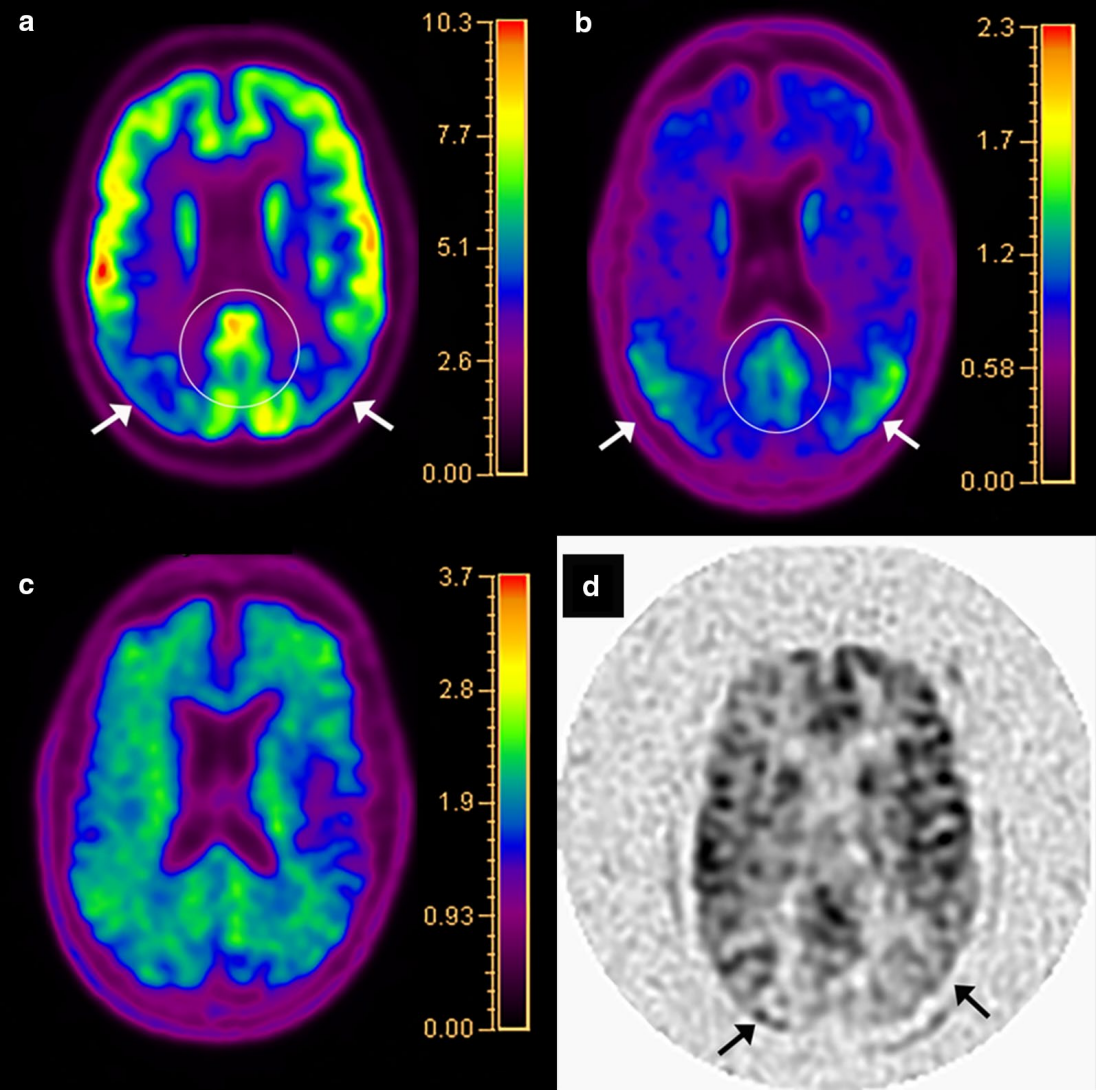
A 73-year-old man with AD, Mini Mental State Examination (MMSE) 23/30. Fluorodeoxyglucose PET/CT (a) demonstrates hypometabolism involving the bilateral parietal lobes (arrows) and precuneus and PCC (oval). Flortaucipir PET/CT (b) shows tau retention in the bilateral parietal lobes (arrows) and precuneus and PCC (oval), mirroring the regions of hypometabolism. Florbetapir PET/CT (c) demonstrates diffuse cortical uptake with “kissing hemispheres.” Arterial spin labeling (d) demonstrates hypoperfusion to the bilateral parietal lobes (arrows), similar to the FDG-PET/CT
